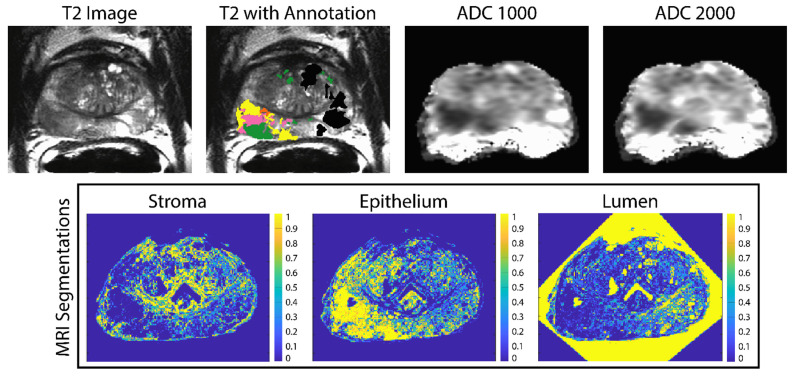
Example of the ADC maps and pathologist annotations aligned to the T2-weighted image (top). The annotation key is as follows: G4FG (yellow) G4CG (pink), G3 (green) and benign atrophy (black). The stroma epithelium and lumen segmentations shown on the (bottom) are likewise aligned to the T2.
